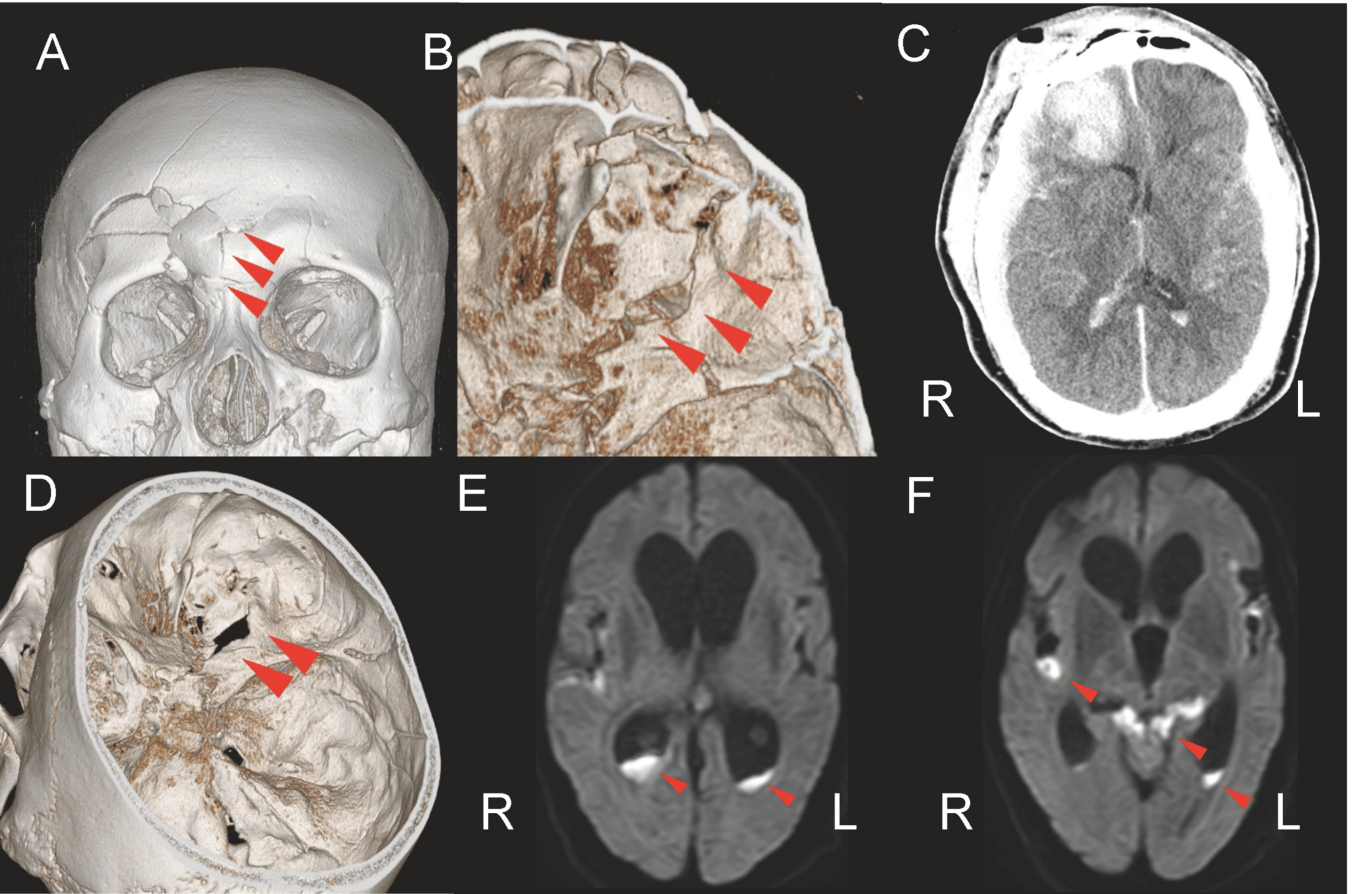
Imaging findings in head trauma and delayed onset meningitis. A: Frontal view of the initial three-dimensional CT image at the time of head trauma. Red arrows indicate the fracture site. B: Superior view of the right frontal skull based on 3D CT scan images at the time of head trauma. The red arrows indicate the fracture line and bony defect. C: Axial CT tomography following head trauma showing an intraparenchymal hematoma within the right frontal lobe contusion. D: 3D CT image at the start of meningitis treatment, showing the view from above the skull base. E: Axial DWI image at meningitis onset. Red arrows indicate the high-intensity areas in both of the lateral ventricles. F: Axial DWI image at the onset of meningitis. Red arrows indicate high-intensity areas within the ventricles and subarachnoid spaces. DWI: diffusion-weighted imaging
